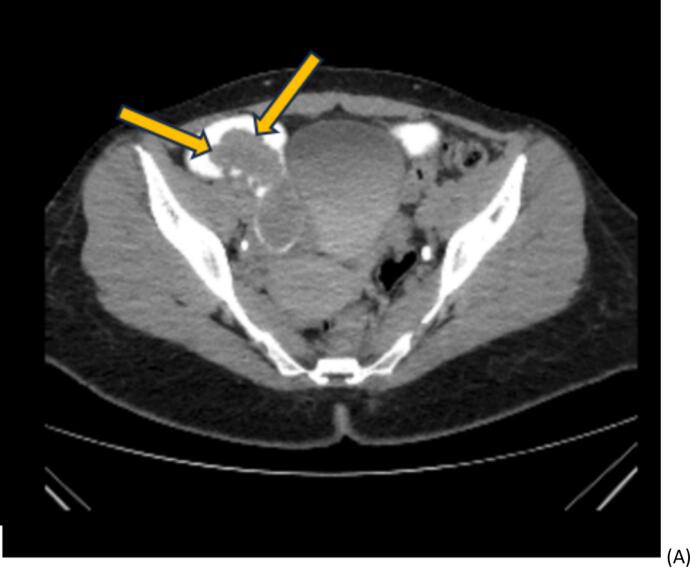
Contrast-enhanced CT scans demonstrating a tumor in the right quadrant of the abdomen with the characteristic bowel-within-bowel configuration suggestive of intussusception (A: axial view; B: coronal view, arrows).
